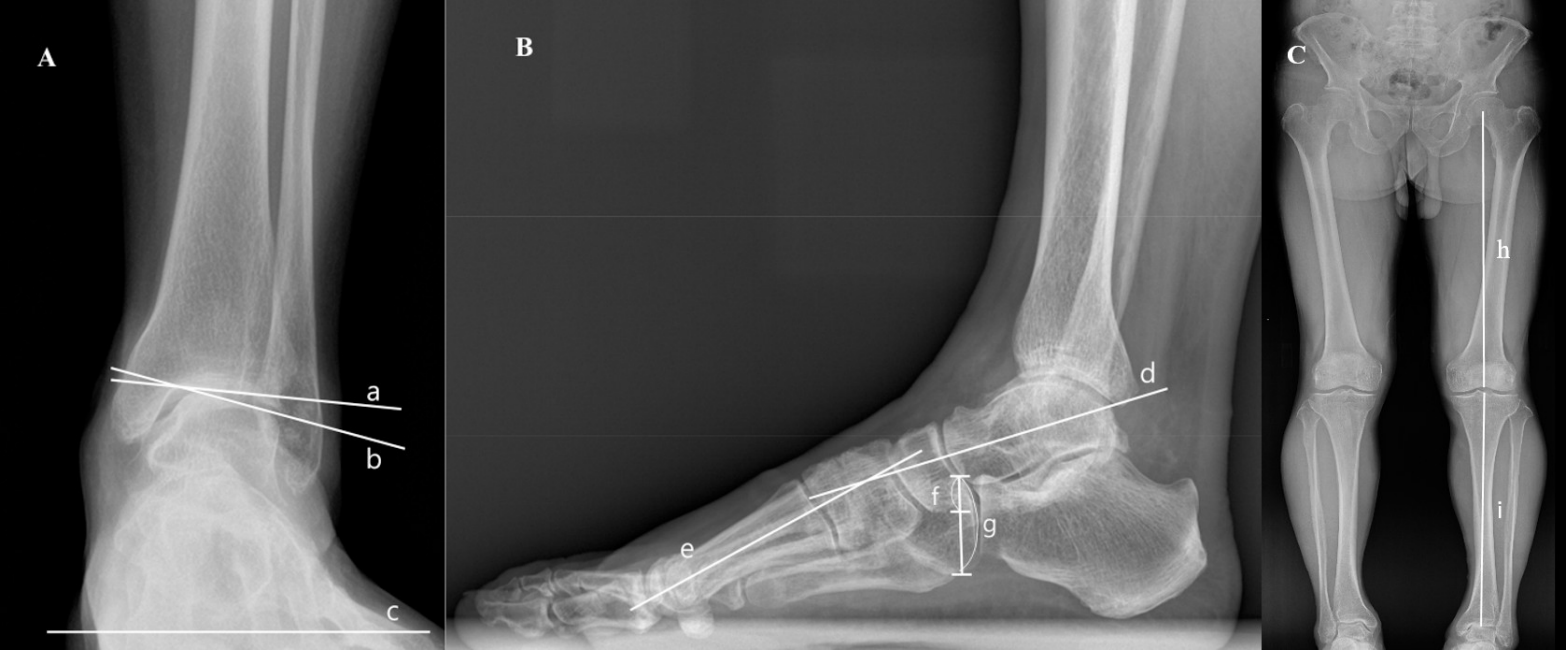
Radiographic measurements. A: The ankle in AP view, TPI is the angle between the tibial plafond (a) and the floor (c). The TT is measured between the tibial plafond (a) and the talar dome (b). B: The foot and ankle in lateral view, the lateral talo-first metatarsal angle is the angle between the longitudinal axis of the talus (d) and the first metatarsal (e). The naviculo-cuboid overlap is the overlapped portion between the navicular and cuboid (f) divided by the vertical height of the cuboid (g). C: The mechanical tibiofemoral angle is the angle between the mechanical axis of the femur (h) and that of the tibia (i).
